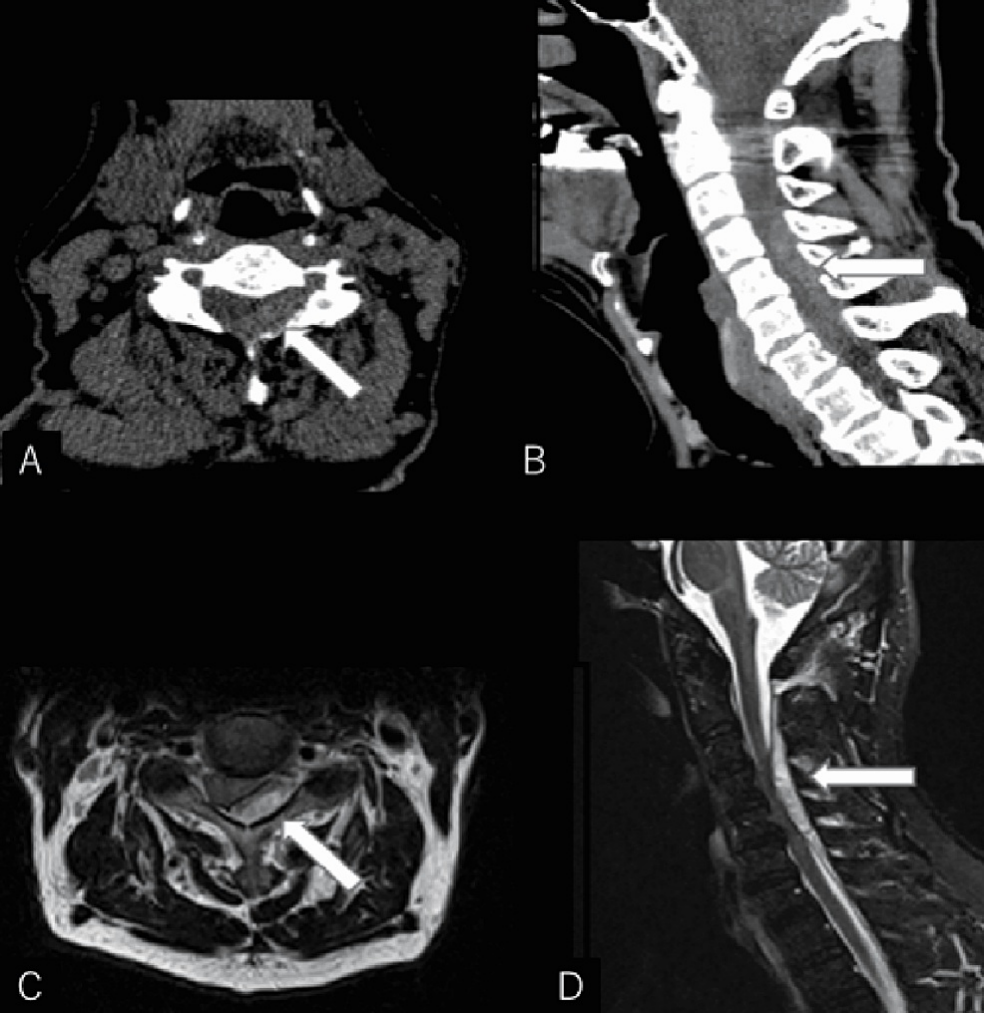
Case 2 preoperative images (A, B) Preoperative cervical CT scan at the C4 level in axial (A) and sagittal (B) views. (C, D) Preoperative cervical T2-weighted MRI images at the C4 level in axial (C) and sagittal (D) views. These show epidural hematoma on the left dorsal side at C3-C6 level.
